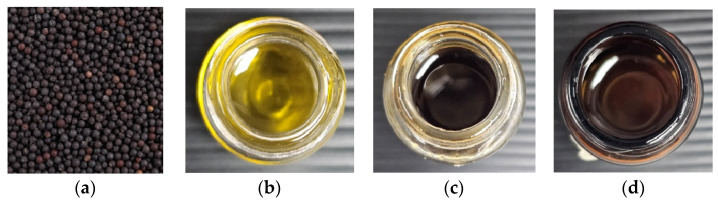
Physical appearance of (a) black mustard seeds, (b) hexane extract (HE), (c) ethanolic extract obtained from Soxhlet extraction (EE), and (d) ethanolic extract obtained from ultrasonication-assisted extraction (UE).
